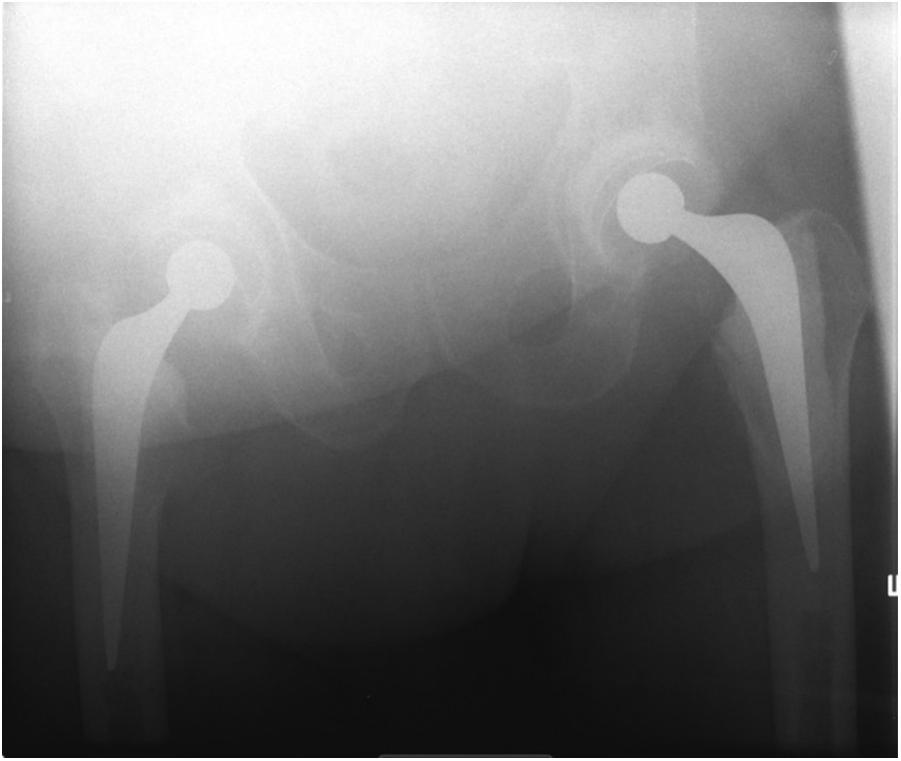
Postoperative X-ray after stem revision.
